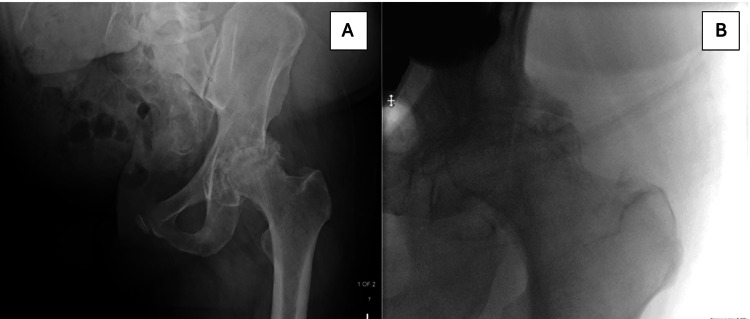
Preoperative X-ray of the left hip. A: Lateral view of the left hip joint showing osteoarthritic changes. B: Anterior view of the left hip joint showing osteoarthritic changes.
